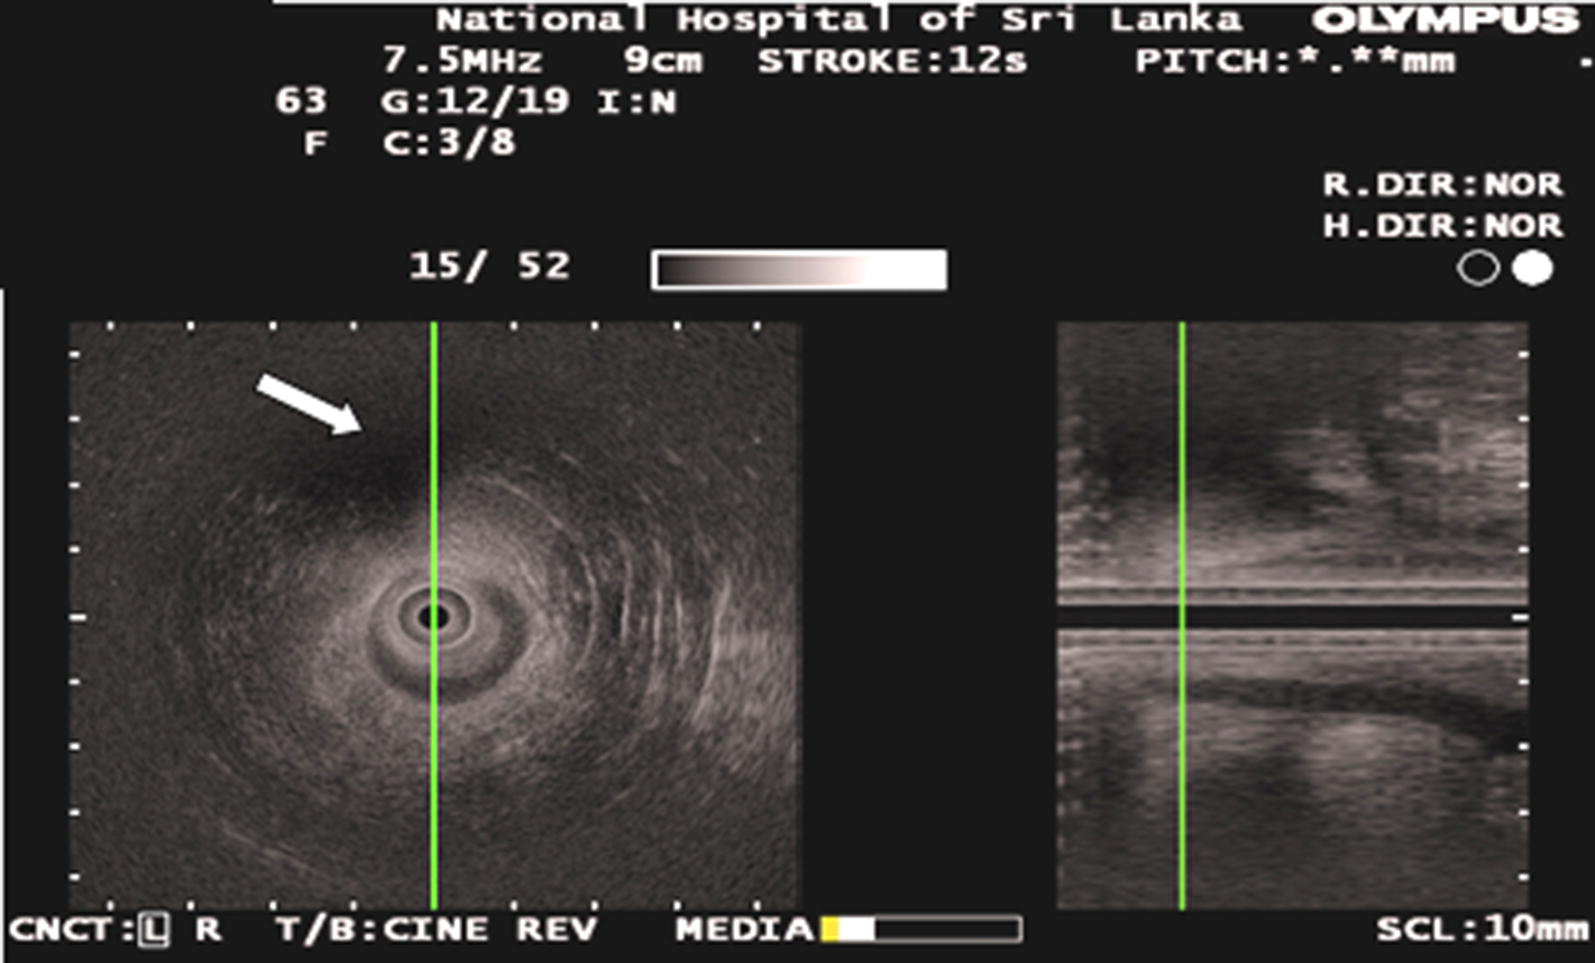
Transverse and sagittal anal endosonogram obtained with a 7.5 MHz transducer at the middle anal canal level shows a transphincteric track (arrow)
